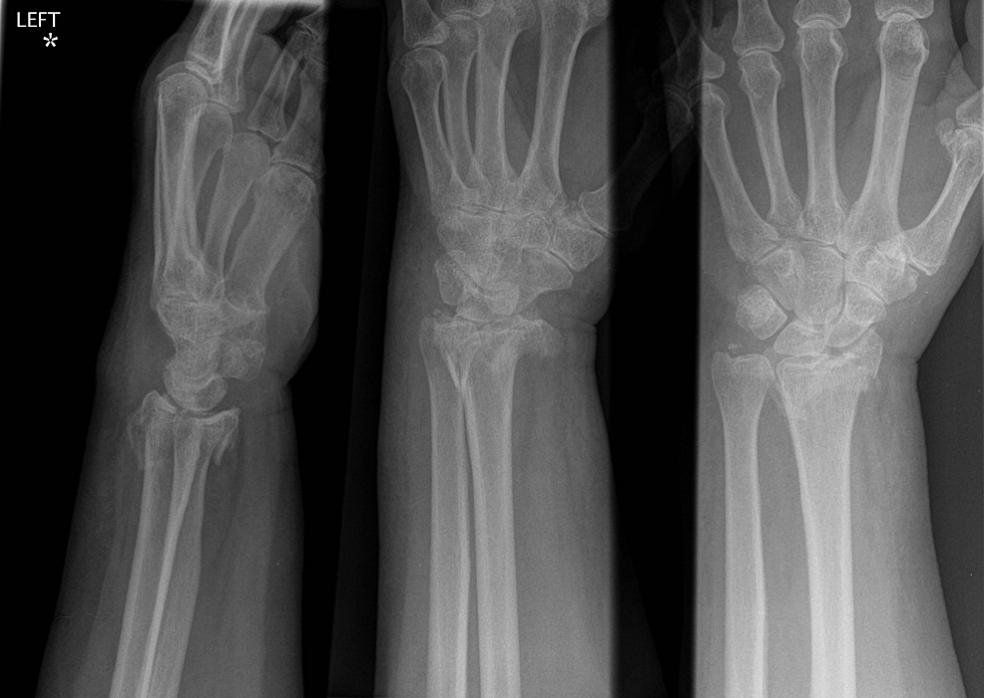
Preoperative radiographs of a patient whose fracture was fixed with the Aptus® osteotomy plate
